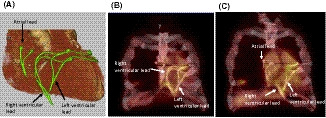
A, Three‐dimensional computed tomographic image of the whole heart. Atrial, right ventricular, and left ventricular leads were implanted on the epicardium. B,C, Coronal views of ^18^F‐FDG‐PET showed local increase in ^18^F‐FDG accumulation in the atrial, right ventricular, and left ventricular leads. Maximum standardized uptake value was 2.5 in the atrial lead, 3.6 in the right ventricular lead, and 5.0 in the left ventricular lead, respectively
